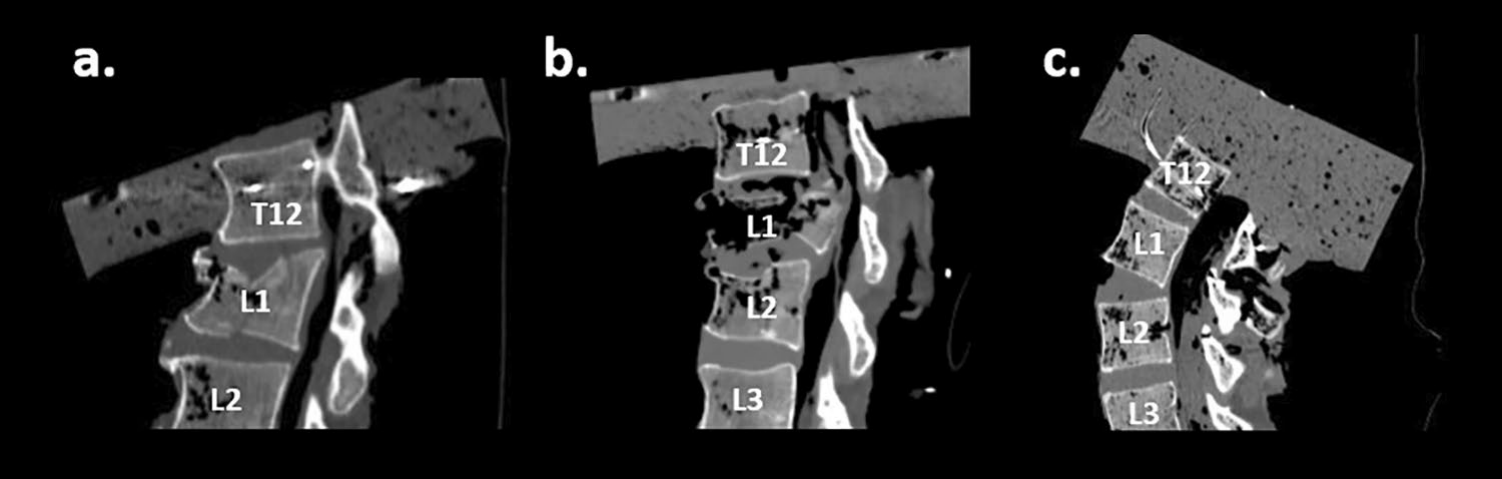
Examples of a wedge, b burst, and c hyperextension fractures from post-test CT scans
